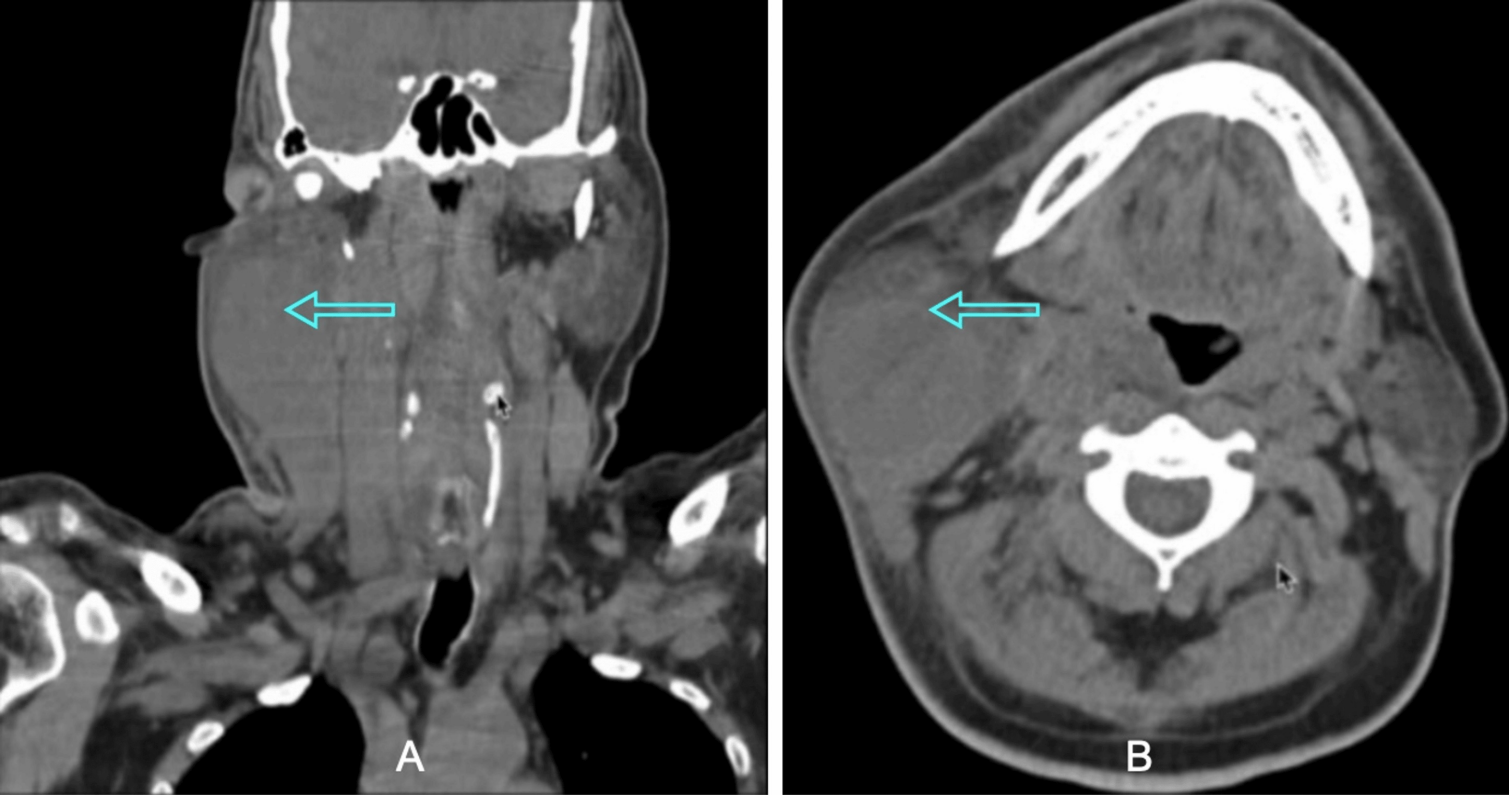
A- Coronal View; B- Axial View. Both panels A and B show CT neck soft tissue without contrast showing right-sided Ludwig angina.
